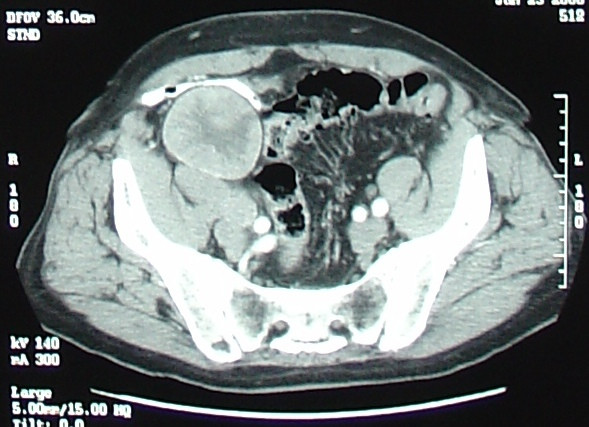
CT scan of pelvis showing right lower quadrant mass.
